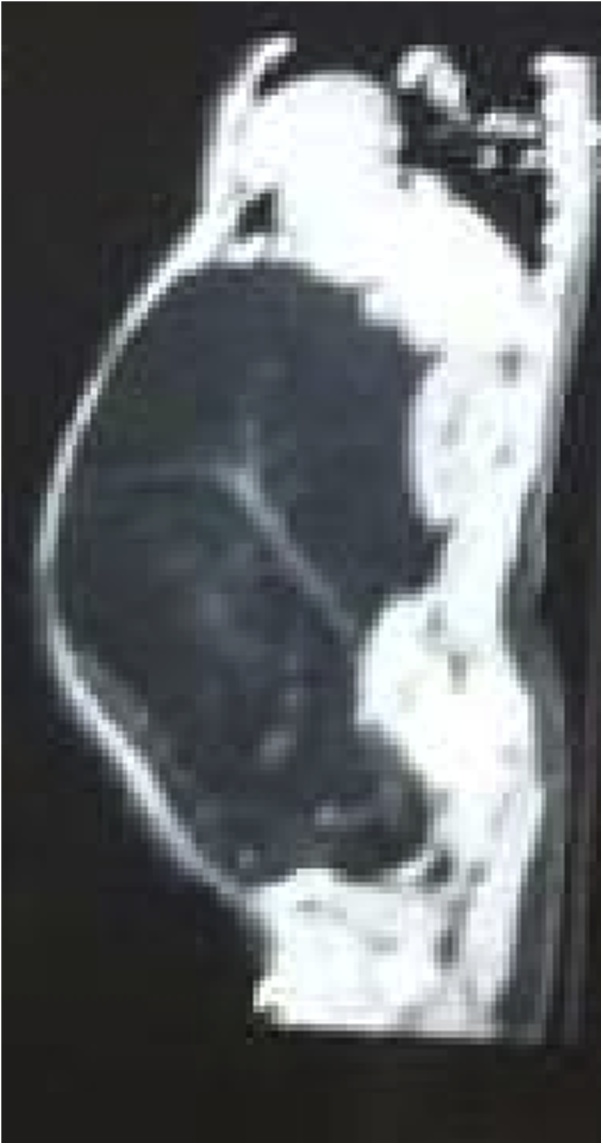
Sagittal computed tomography scan showing fat density mass occupying all abdominal cavity.
